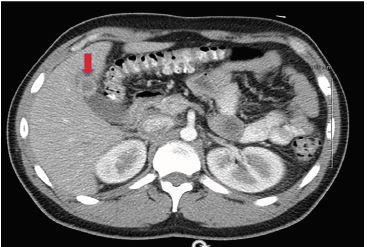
Computed tomography demonstrating gallbladder wall thickening (red arrow).
